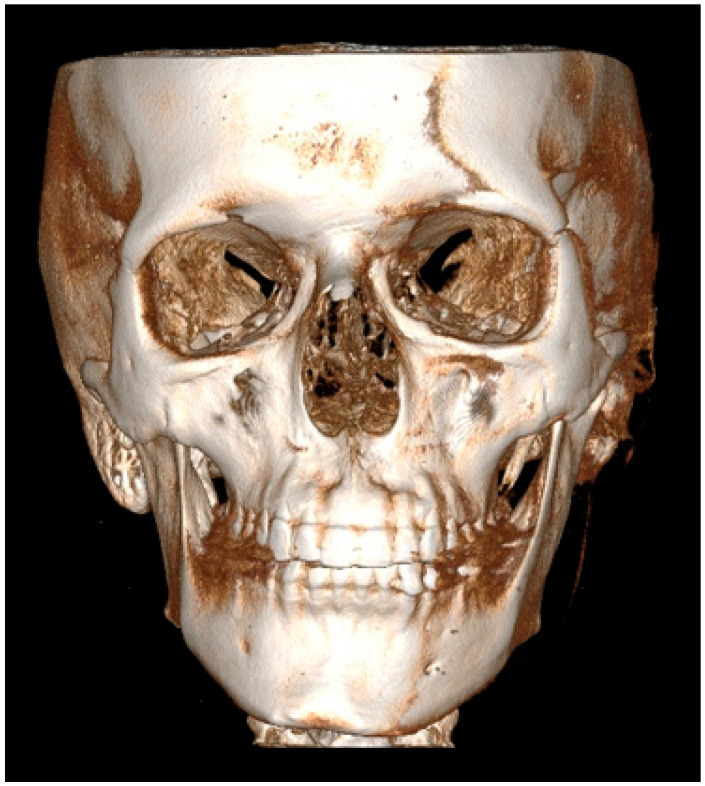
CBCT 3d-evaluation in left-sided mandibular condylar hyperplasia (CH)—an asymmetry noticed in the mandibular left body and chin position followed by deviation in the maxillary cant.
